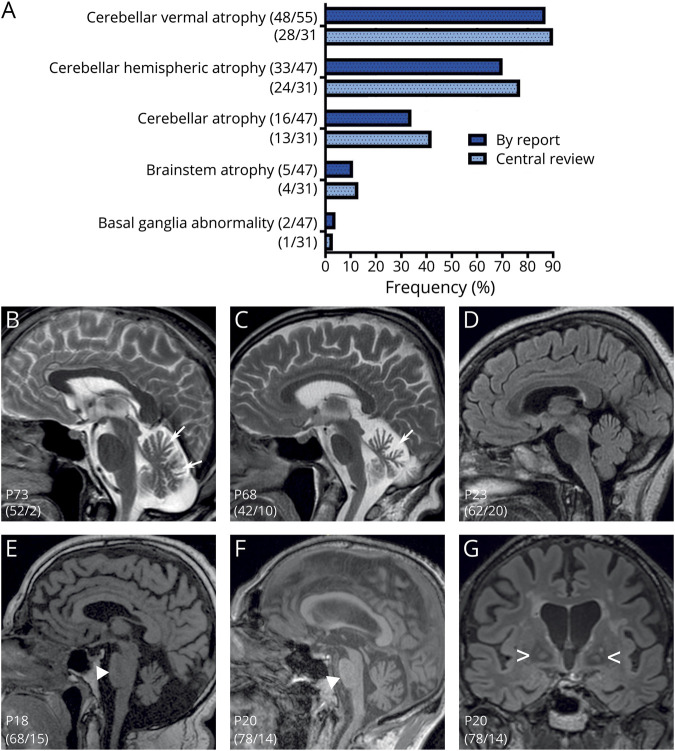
MRI Features of RFC1 Disease (A) MRI findings by report and by centralized review of digital images by 2 independent raters. Numerator and denominator in brackets indicate the number of patients with a feature and the number of patients assessed for this feature, respectively. (B–D) Mild to moderate cerebellar atrophy of the vermis with variable extent and temporal evolution, for example, marked cerebellar atrophy at 42 years of age in P68 vs absence of atrophy in P23 at 62 years of age after 20 years of ataxia. (E and F) Representative images of mild pontine atrophy after >14 years’ disease duration. (G) Patient with pallidal T2 signal abnormalities. Numerator and denominator in brackets on images indicate age and ataxia duration at MRI. RFC1 = replication factor complex subunit 1.
